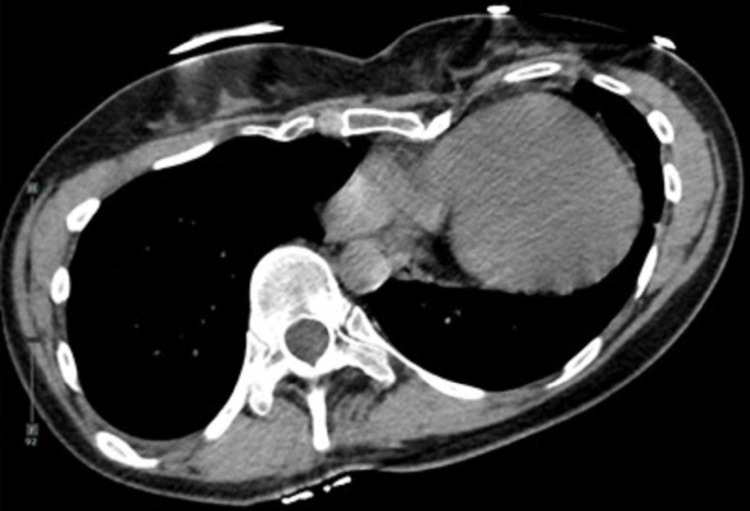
Preoperative chest computed tomography showing significant pectus deformity causing leftward cardiac displacement and direct cardiac compression with subsequent left lung compression.
